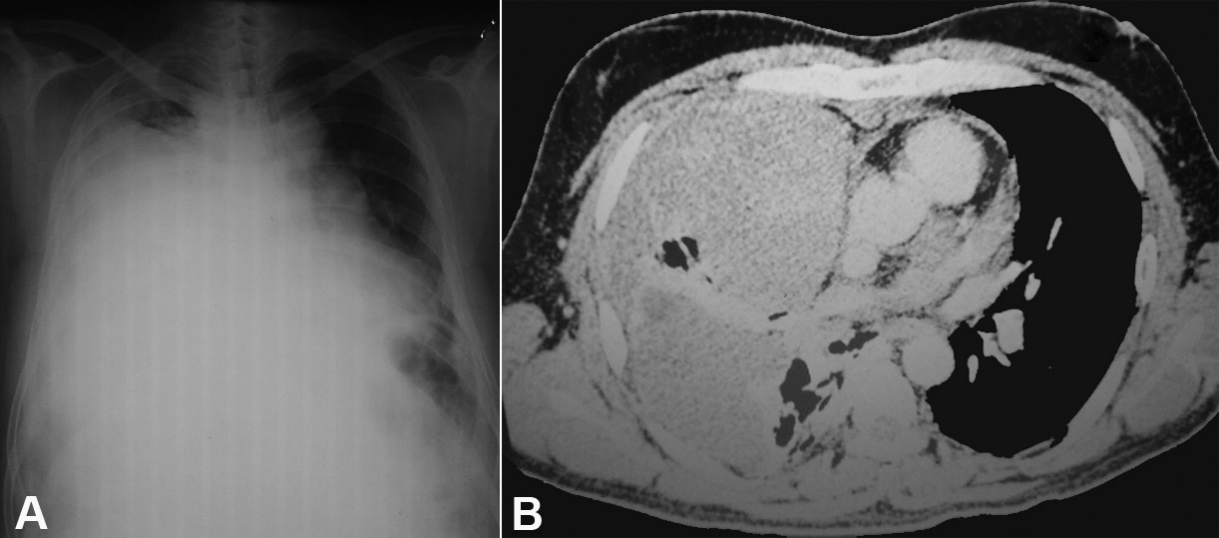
A – Chest X-ray showing a homogenous opacity in right hemithorax, and contralateral mediastinal shift; B – Thoracic CT showing a pleural based large non-enhancing mass reaching the chest wall anteriorly. Note the preservation of the fat planes. Posteriorly the mass abuts and distorts the right upper lobe bronchus, stretches the ascending branch of right superior pulmonary vein, medially abuts the superior vena cava in its distal course and the ascending aorta at its proximal course and superiorly displaces the horizontal fissure causing the collapse of the middle lobe.
